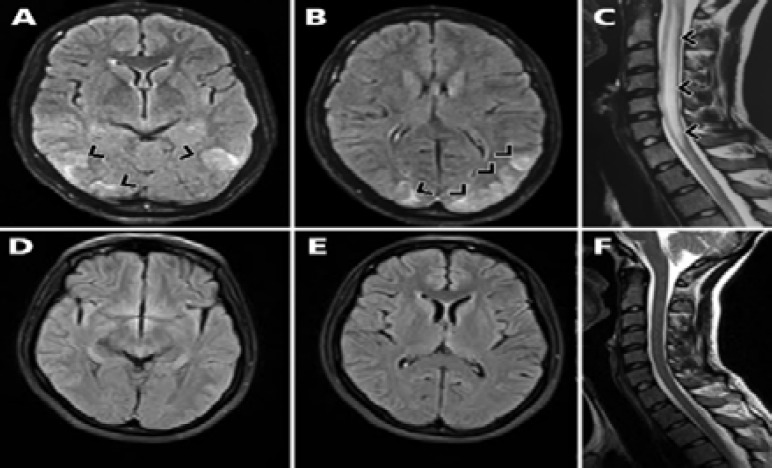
Axial fluid-attenuated inversion recovery (FLAIR) brain magnetic resonance imaging (MRI) indicating hypersignal lesions in parieto-occipital areas in favor of posterior reversible encephalopathy syndrome (PRES) at admission (A, B); sagittal T2 cervical MRI demonstrating a longitudinally extensive lesion in the spinal cord at admission (C); two weeks later, the hyperintense signals had completely disappeared on the brain and spinal cord MRIs (D-F).
